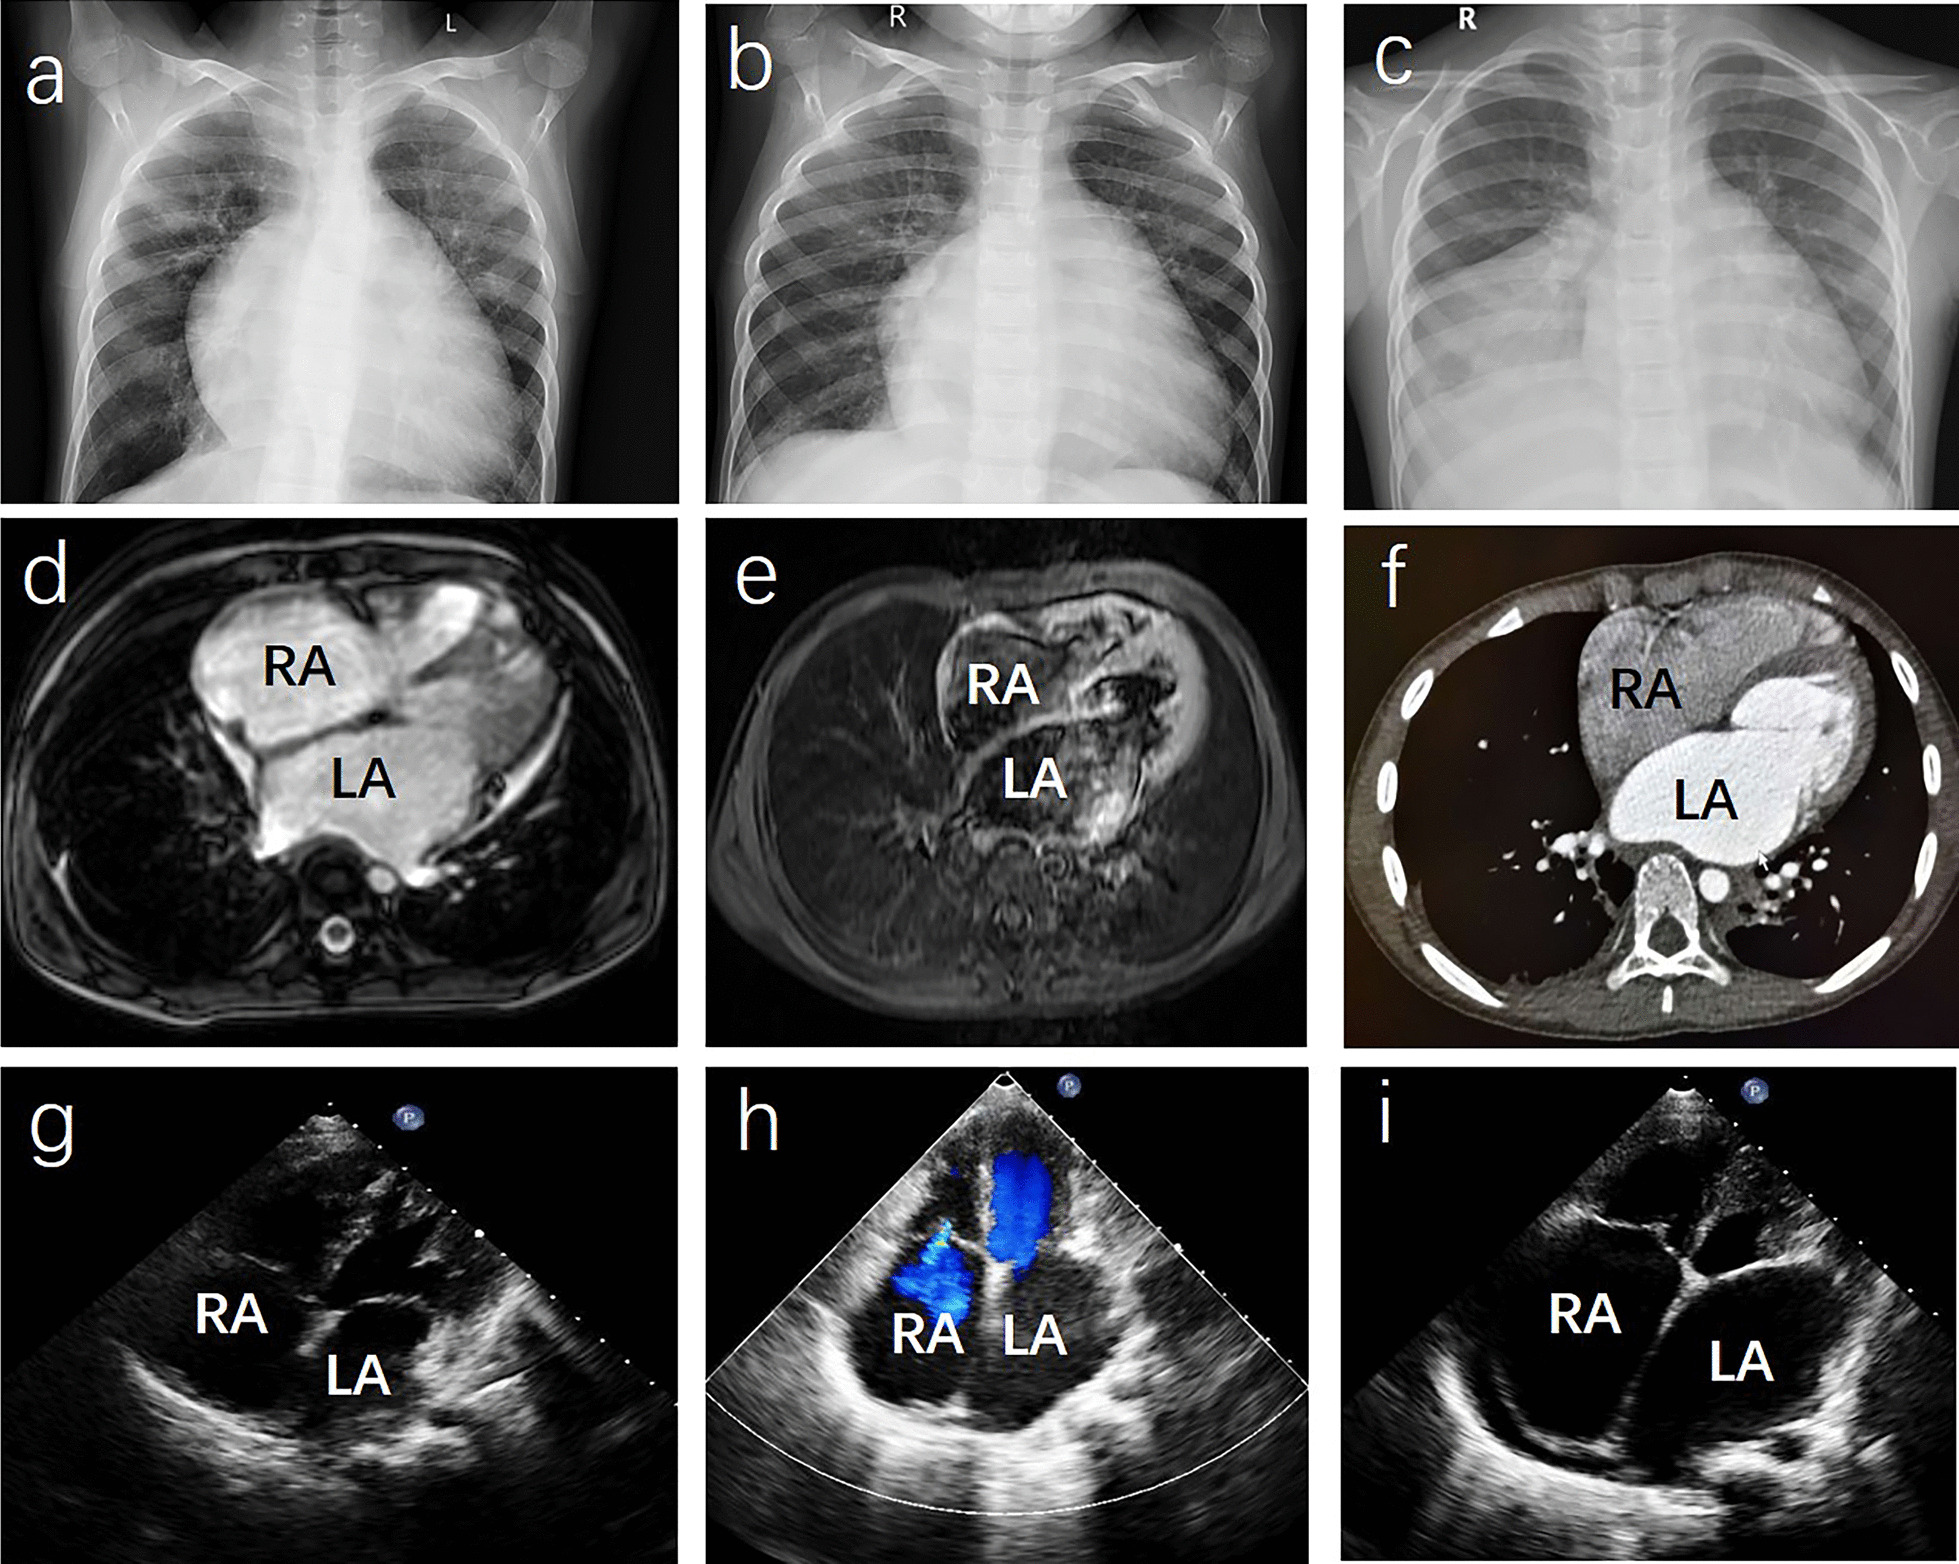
Representative images of cardiac X-ray, MRI, CTA, and two-dimensional echocardiography. *LA* left atrium, *RA* right atrium. a (case 1), b (case 2), c (case 4): Chest X-ray showed increased heart shadow without calcification of the pericardium, thickened pericardium, or endocardium. d (case 2), e (case 4): Cardiac MRI showed bi-atrial enlargement without calcification of the pericardium, thickened pericardium, or endocardium. f (case 4): Cardiac CTA showed bi-atrial enlargement without calcification of the pericardium, thickened pericardium, or endocardium. g (case 1), h (case 2), i (case4): Two-dimensional echocardiography showed dilation of the left and right atria with normal ventricular sizes, without calcification of the pericardium, thickened pericardium, or endocardium
